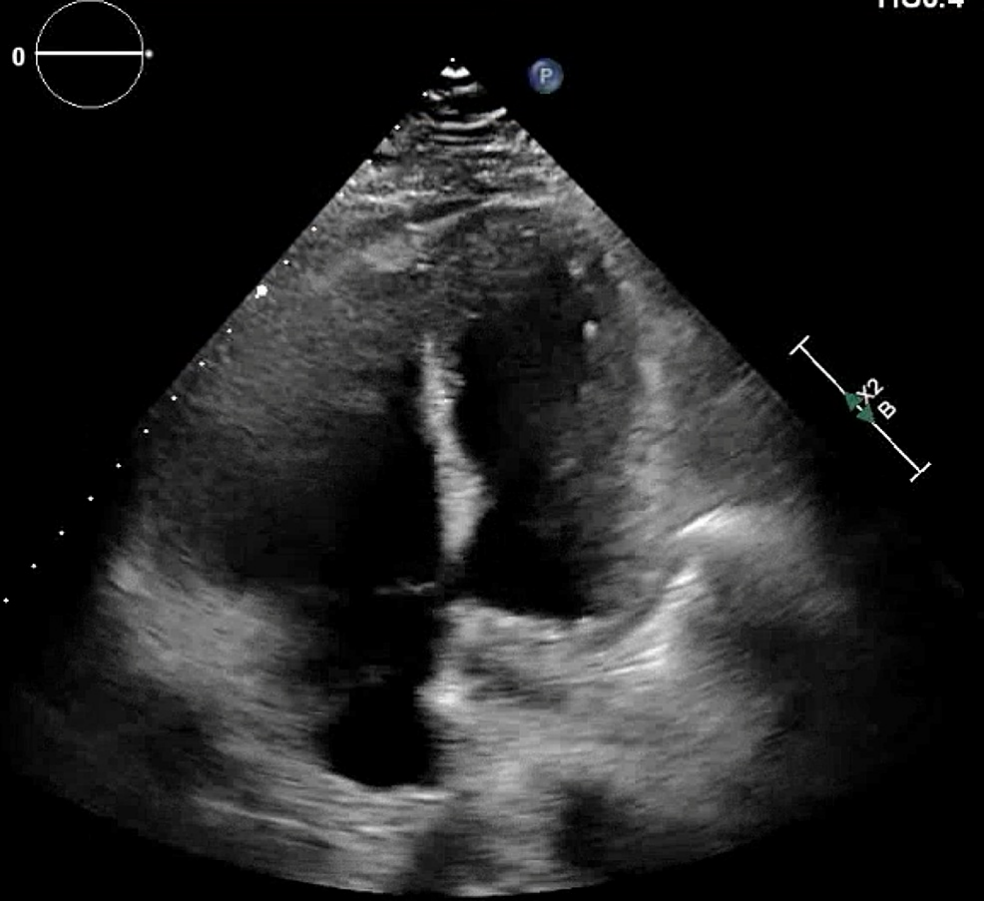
Follow-up transthoracic echocardiogram showing thrombus resolution in the right atrium (apical four-chamber view).
